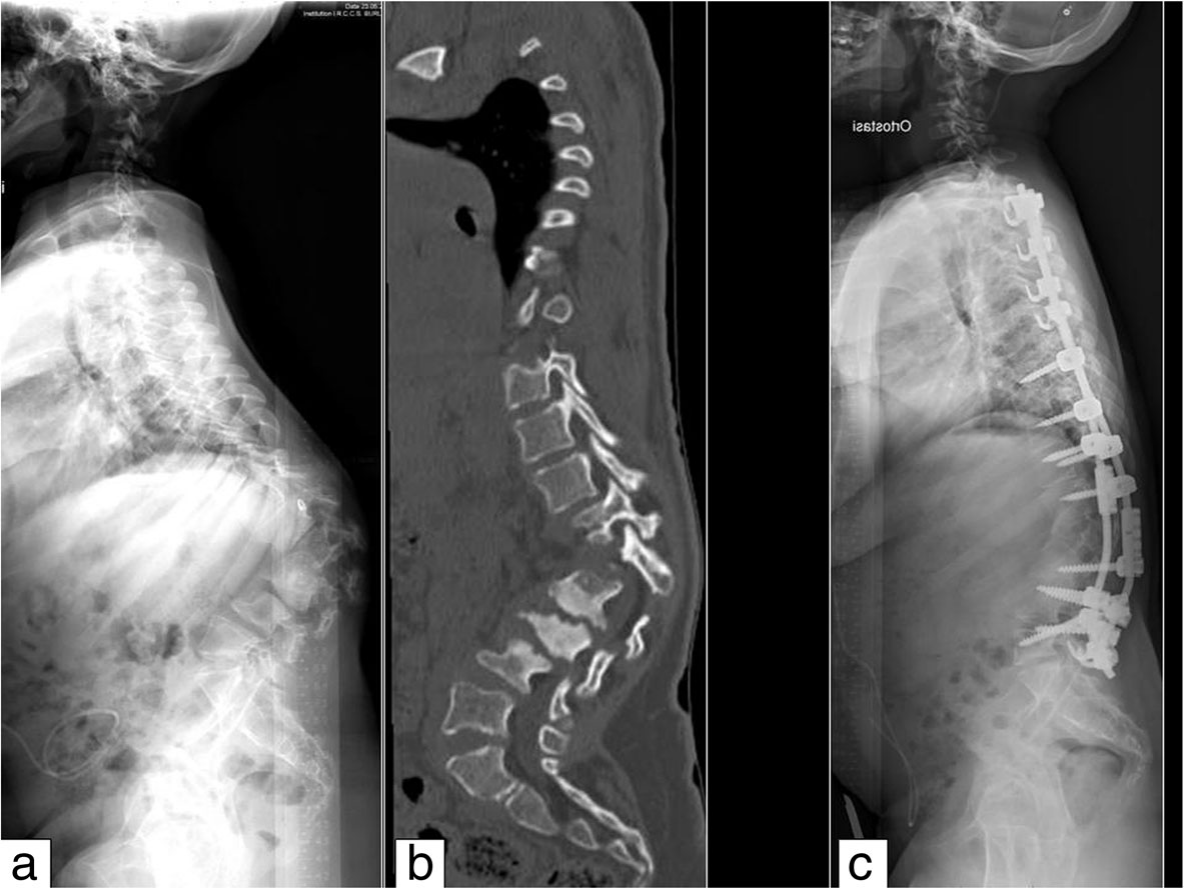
Thoracolumbar kyphosis in a 12-year-old girl with MPS I. a Preoperative lateral x-ray showing severe wedge deformity of L1, with kyphosis of about 90°. b Computed tomography scan shows the dysostosis of the vertebrae. c Postoperative lateral x-ray showing good correction of the kyphosis after anterior fusion with tricortical bone graft and instrumented posterior fusion
